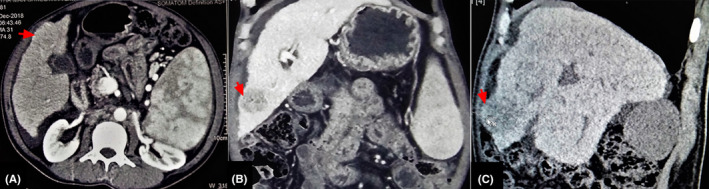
CECT scan of abdomen and pelvis (A—axial, B—coronal, C—sagittal) showing 4.6 × 3 cm well‐circumscribed, hypodense mass in segment IVb of liver in subcapsular region (red arrows). The mass is in contact with anterior abdominal wall with loss of fat plane. CECT, contrast‐enhanced computed tomography
